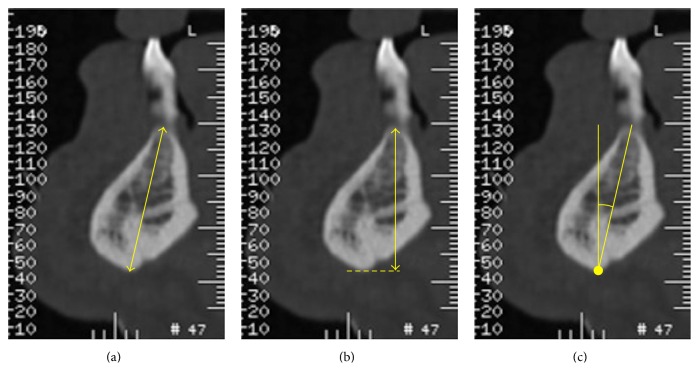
(a) The distance between the most coronal and medial point of the ridge and the lowest point along the axis of the crest; (b) the height from the most coronal and medial point of the ridge to the projection of the lowest point on the perpendicular to the CT reference plane; (c) the angle between the axis of the crest and the line perpendicular to the CT reference plane.
